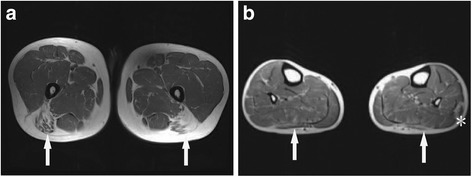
Muscle MRI comprising T1-weighted images of the thigh and lower leg from patient 2. a. Transverse cuts from the mid-thigh muscles showed mild increase in signal intensity within the femoris long head (arrows); b, Transverse cuts from the lower leg showed mild increase in signal intensity within the peroneus longus (arrows) and there was atrophy of gastrocnemius lateral heads (asterisk)
